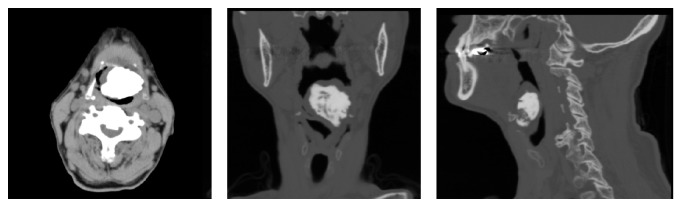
CT scan of the neck. A large osseous mass adjacent to the hyoid bone was observed.
